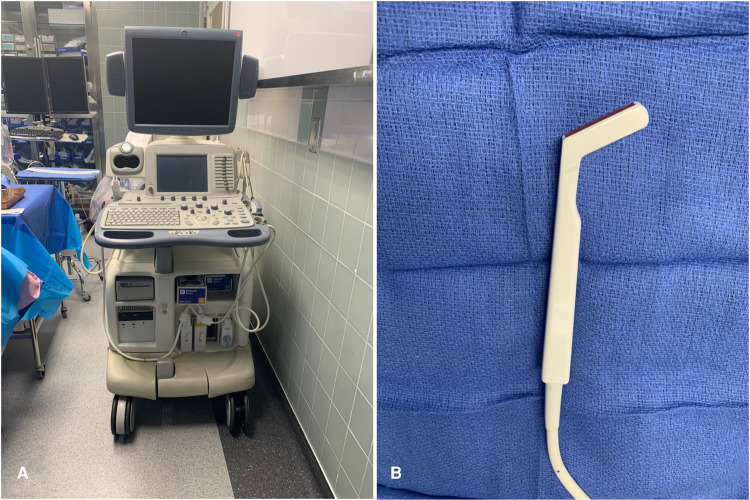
(A) Intra-operative GE ultrasound used for measuring sigmoid sinus velocities. (B) The hockey stick probe used for measuring sigmoid sinus velocities.
